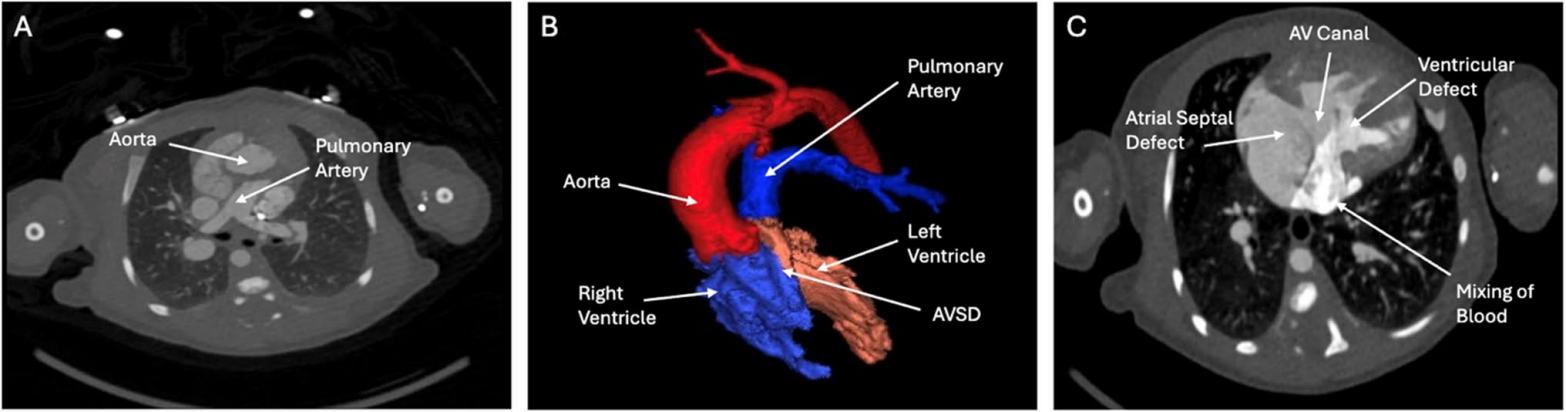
(A) Transverse computed tomography angiography showing discordant ventriculo-arterial connections with an anterior and right-sided aorta. (B) Three-dimensional reconstruction of the heart showing discordant ventriculo-arterial connections with an anterior aorta arising from the right ventricle and a pulmonary artery arising from the left ventricle, consistent with isomerism of the right atrial appendages. (C) Transverse computed tomography angiography showing common atrioventricular canal and atrioventricular septal defect with absence of ventricular septation
